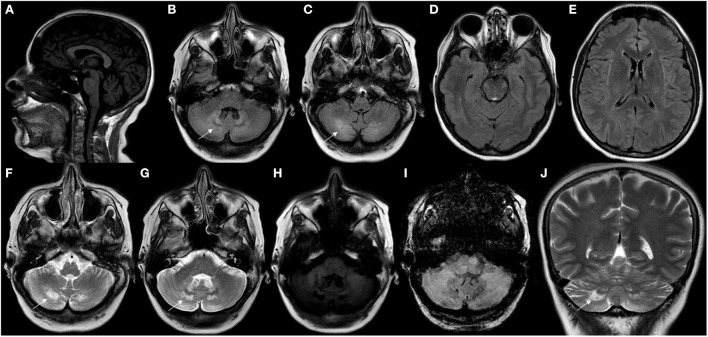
Neuroimaging findings in CTX. (A) Sagittal T1W brain MR imaging (MRI) disclosed mild cerebellar atrophy. (B–G) Axial brain MRI showed hyperintensity involving cerebellar white matter and dentate nuclei (white arrows) in FLAIR (B–E) and T2W imaging (F, G). Axial brain MRI showing mild hypointensity involving cerebellar white matter and dentate nuclei in T1W (H) and SWI sequences (I). Coronal brain MRI showing hyperintensity (white arrow) involving cerebellar white matter and dentate nuclei in T2W imaging (J).
